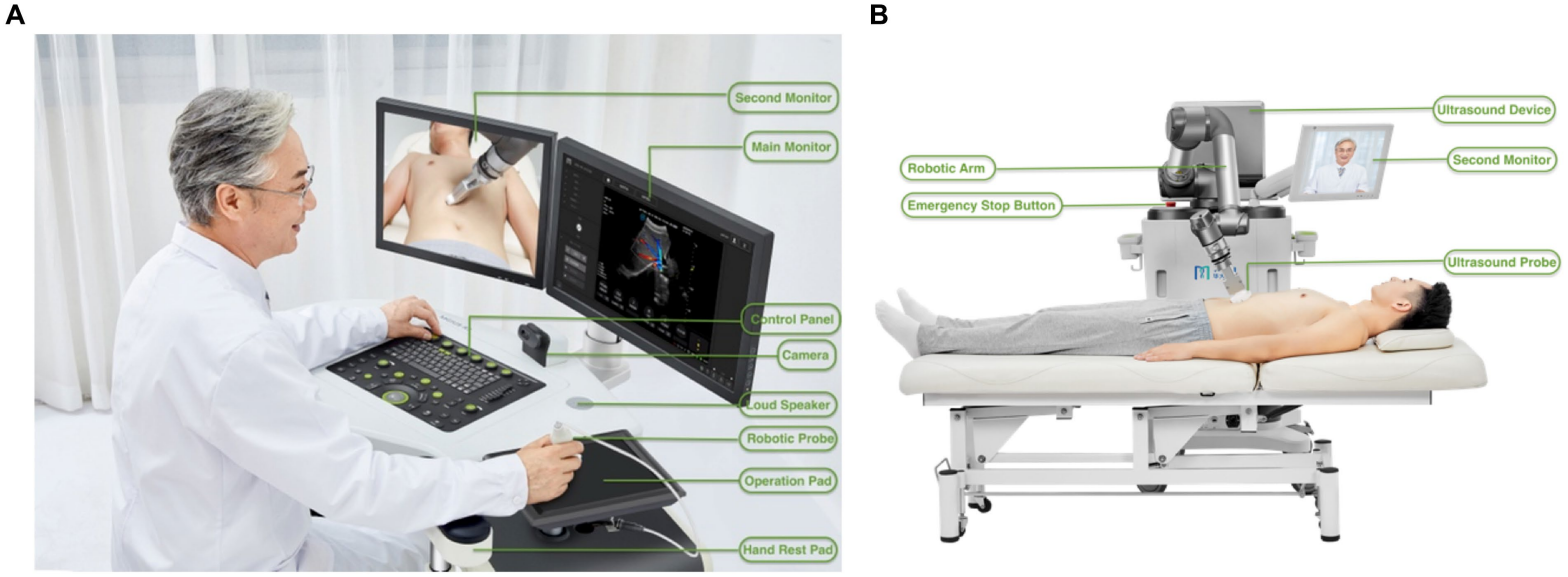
Structural compositions of the robot-assisted teleultrasound diagnostic system. (A) Basic components from the right-front view of the doctor-end system. (B) Basic components from the rear view of the patient-end system. (We acknowledged the pictures provided by MGI Tech Co.)
